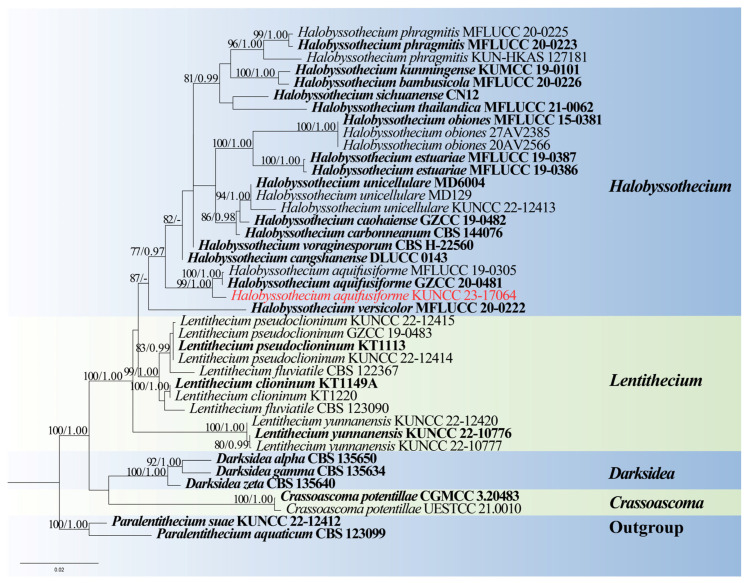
RAxML tree based on analysis of combined ITS, LSU, SSU, and *tef*1-α dataset. The combined analyses include 41 strains with 3416 characters, including gaps (ITS: 576 bp, LSU: 868 bp, SSU: 1020 bp, and *tef*1-α: 952 bp). The tree is rooted to *Paralentithecium aquaticum* (CBS 123099) and *Paralentithecium suae* (CGMCC 3.24265). The tree topology of the maximum likelihood analysis and Bayesian analysis are similar. The RAxML analysis of the combined dataset yielded a best-scoring tree with a final ML likelihood value of −10,581.198409. The matrix had 766 distinct alignment patterns, with 21.26% undetermined characters or gaps. Estimated base frequencies were as follows: A = 0.237748, C = 0.250655, G = 0.270916, and T = 0.240681; substitution rates AC = 0.899013, AG = 1.911393, AT = 0.973835, CG = 1.058482, CT = 5.445001, and GT = 1.000000; and gamma distribution shape parameter α = 0.020000. Bootstrap values for maximum likelihood (ML) ≥ 75% and clade credibility values ≥ 0.95 from Bayesian-inference analysis are labeled on the nodes. The ex-type strains are in bold. The newly obtained sequences are indicated in red.
